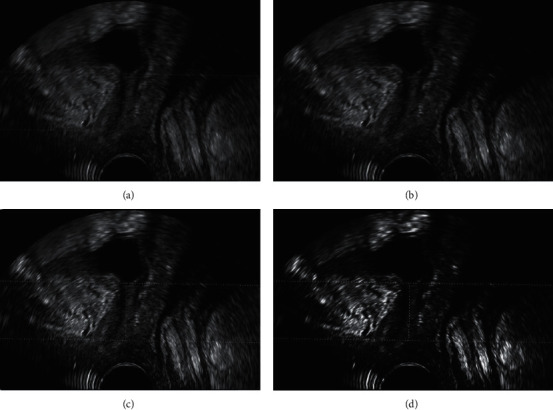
Ultrasound imaging of pelvic floor under various algorithms. (a) SCB algorithm. (b) SER algorithm. (c) RLS algorithm. (d) ISCB algorithm.
